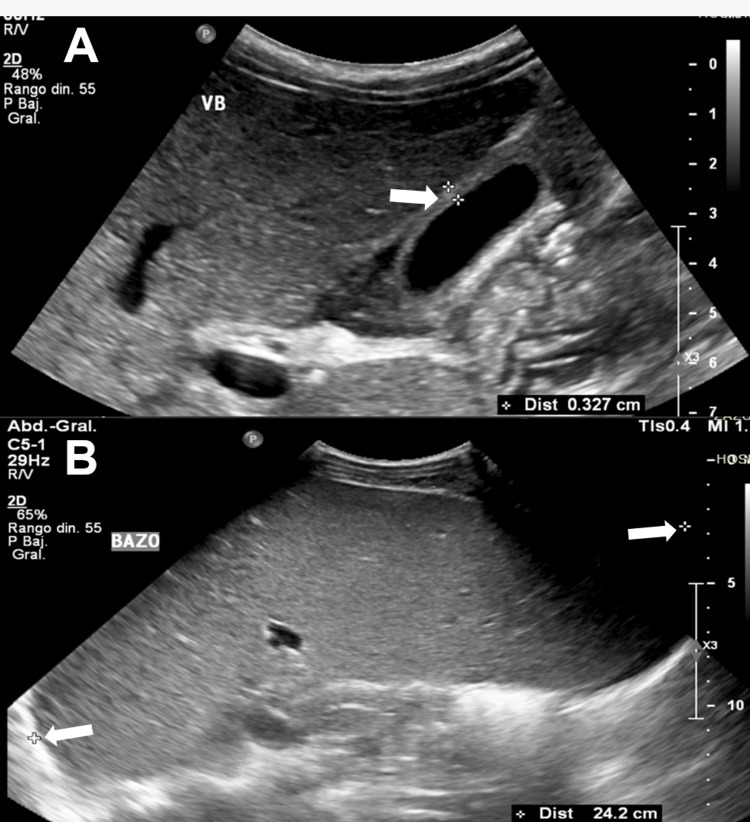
Abdominal ultrasound showing the patient’s gallbladder and spleen. The presence of reactive gallbladder wall edema is observed (arrow in A), as well as marked splenomegaly (arrows in B).
